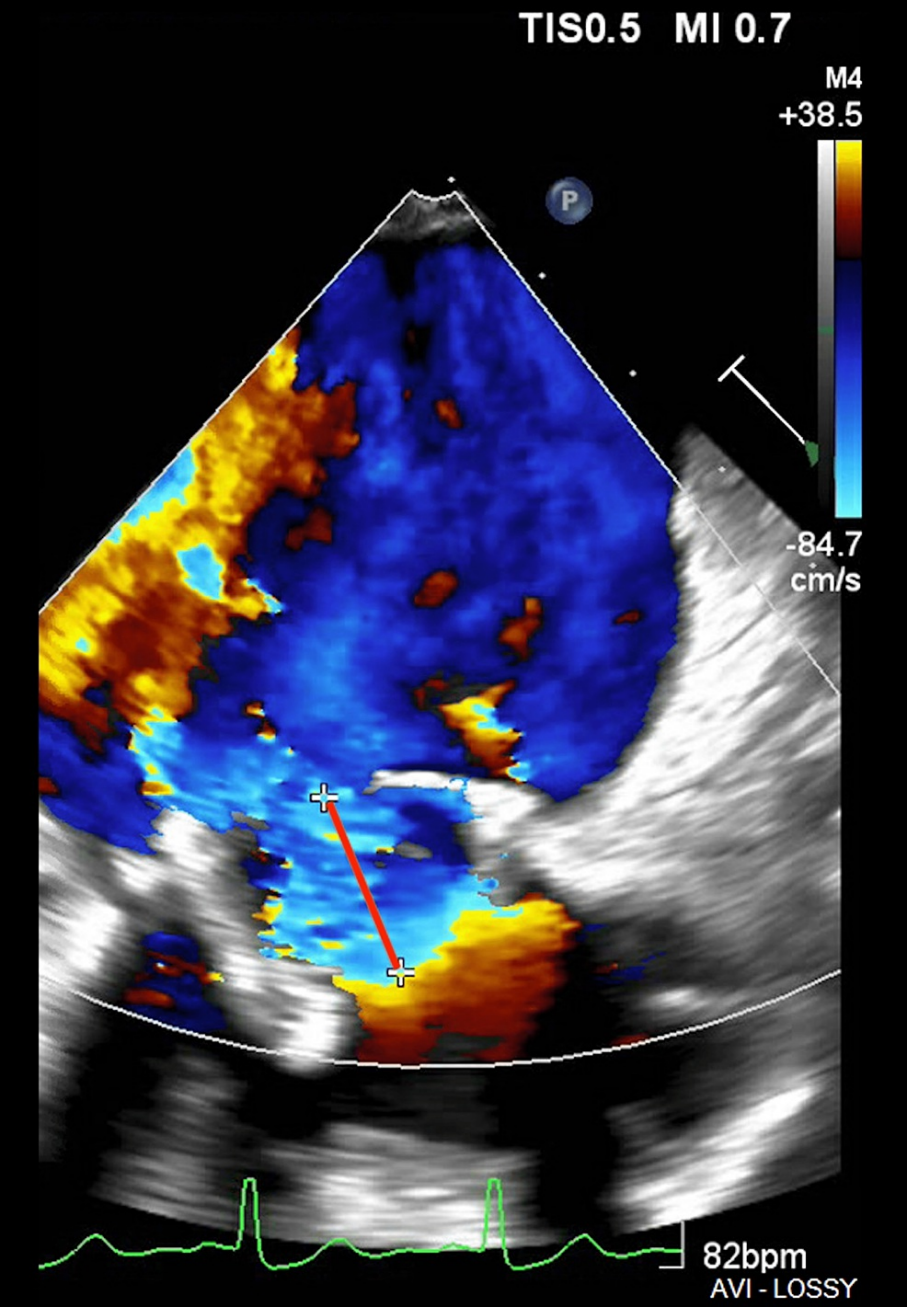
Color Doppler of the severe mitral regurgitation demonstrating a proximal isovelocity surface area (PISA) of 1.2cm (red line).
